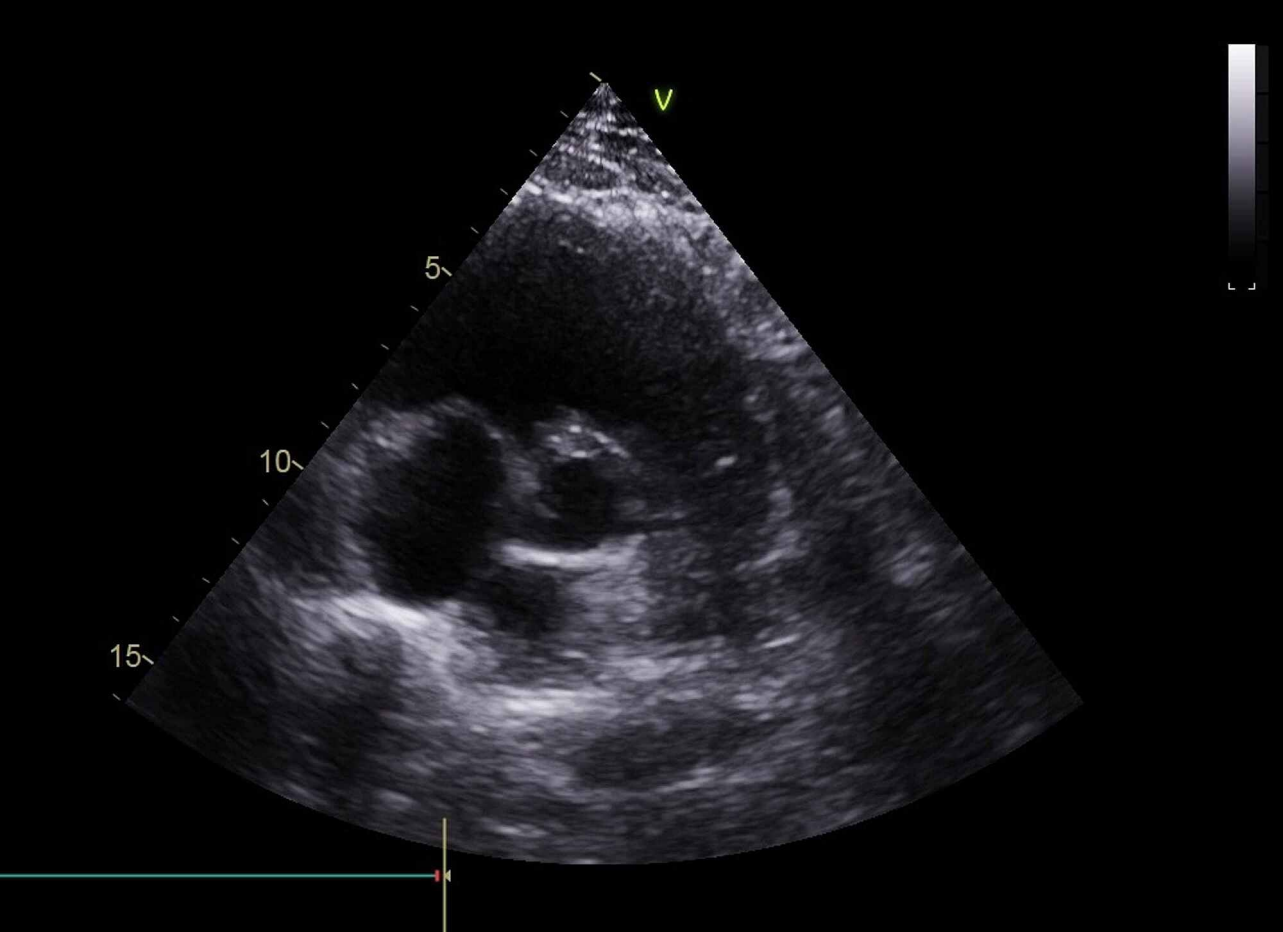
Parasternal short-axis view showing the pulmonary valve and main pulmonary artery
